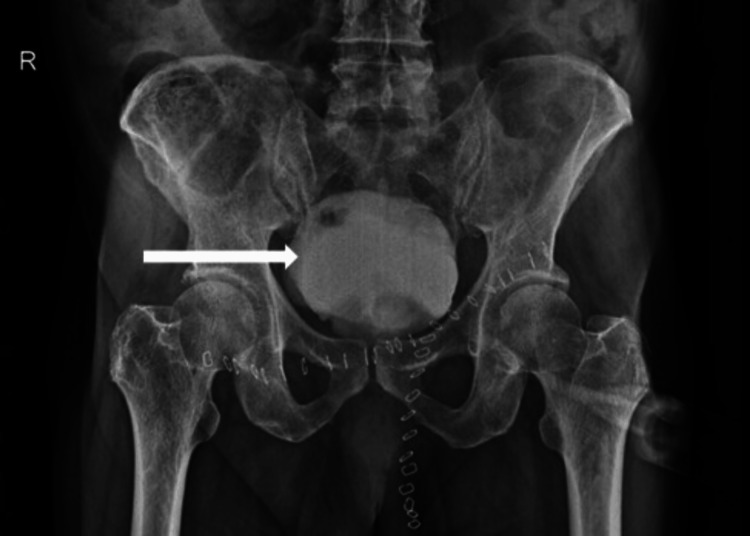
Postoperative X-ray cystogram shows contrast filling in the bladder with no evidence of any contrast leak (long white arrow)
